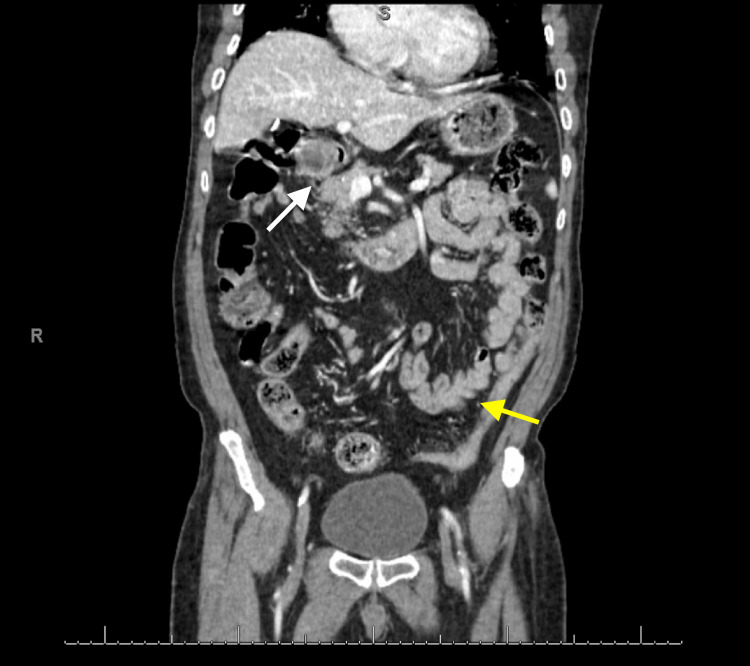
Initial CT scan on admission indicating distal colitis and gastroduodenitis. The white arrow indicates gastroduodenitis and the yellow arrow indicates the distal colitis.
